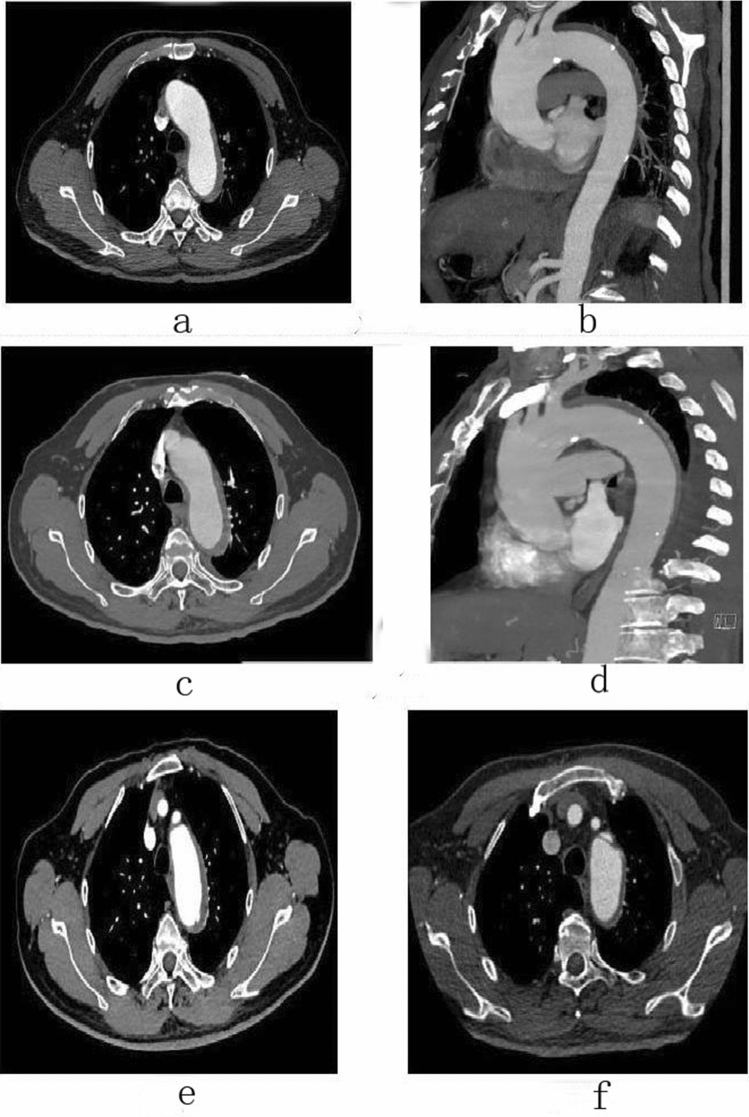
Male, 67 years old, admitted to hospital for “sudden pain in chest for 9 h”, Diagnosis: Stanford type B aortic dissection; Treatment: IMH conservative treatment. CTA of the patient in different periods: (a) Admission; (b) Admission; (c) 3 days after admission; (d) 3 days after admission; (e) 3 months after admission; (f) 11 months after admission.
